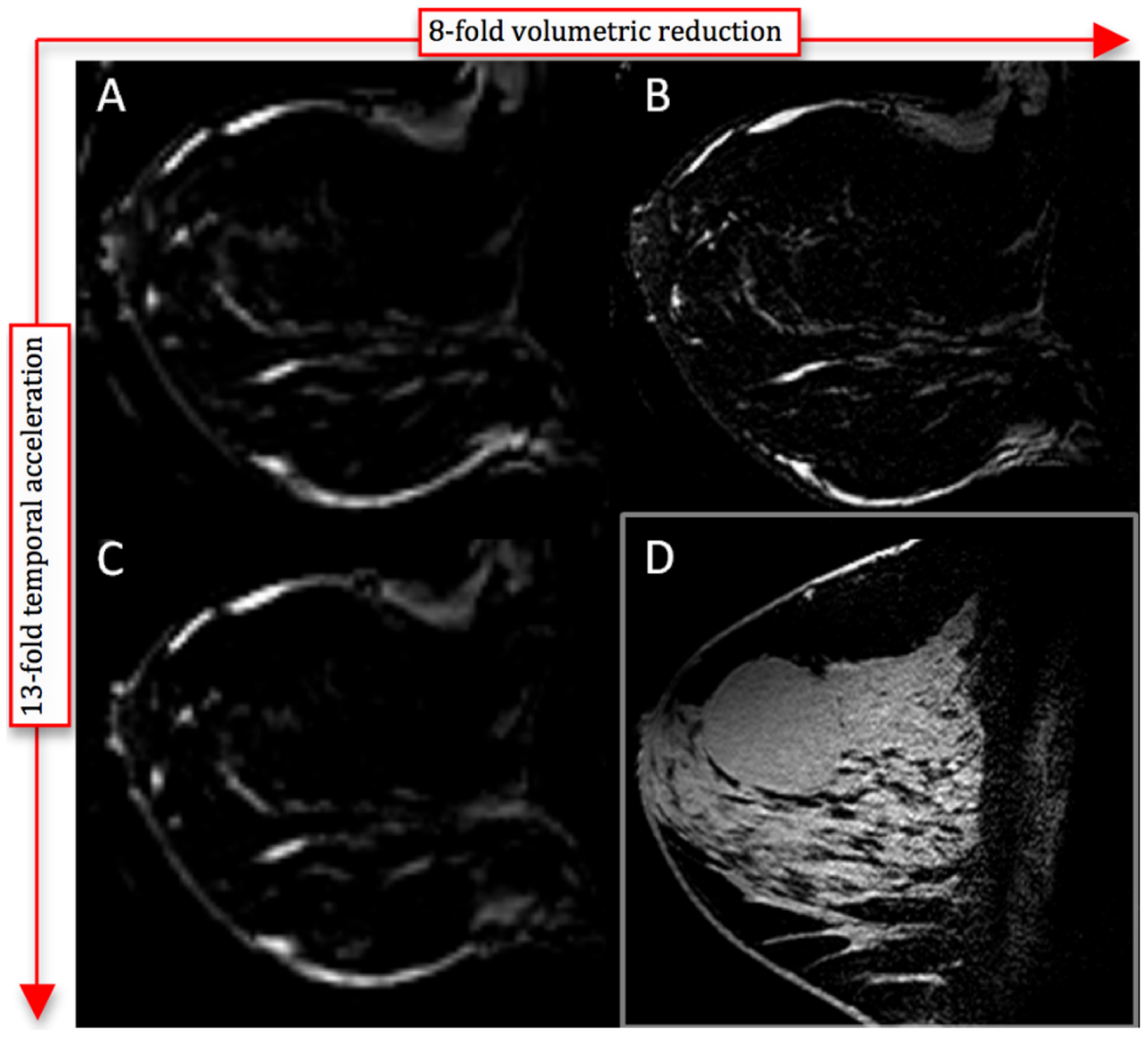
Increased spatial/temporal resolution in *in vivo* breast imaging (3D T1-weighted THRIVE with SPAIR fat suppression). A) Clinically standard 1-mm isotropic resolution with 90 s acquisition time from the same volunteer as Figs. 7 and 8; B) Higher spatial resolution: SENSE accelerated acquisition (2.2 F/H, 2.1 L/R) with 0.5 mm isotropic resolution with 90 s acquisition time, same volunteer as in (A); C) Higher temporal resolution: Ultrafast 7 s acquisition time with 1-mm isotropic resolution with SENSE factor 3×3 [F/H×L/R], same volunteer as in (A); D) High resolution image of a fibroadenoma (0.6-mm isotropic, SENSE 2×2, 60 s acquisition) in the right breast of a patient.
